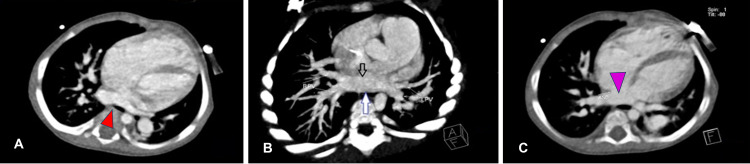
Contrast cardiac CT scan (axial view) of the 11-month-old boy (A) Axial image showing membrane of cor triatriatum (red arrowhead), left atrium, and ASD. (B) Axial maximum intensity projection showing membrane of cor triatriatum (black arrow) and posterior wall of cor triatriatum (blue arrow). (C) Large ASD between right and left atria (purple arrowhead). Abbreviations: CT, computed tomography; ASD, atrial septal defect.
